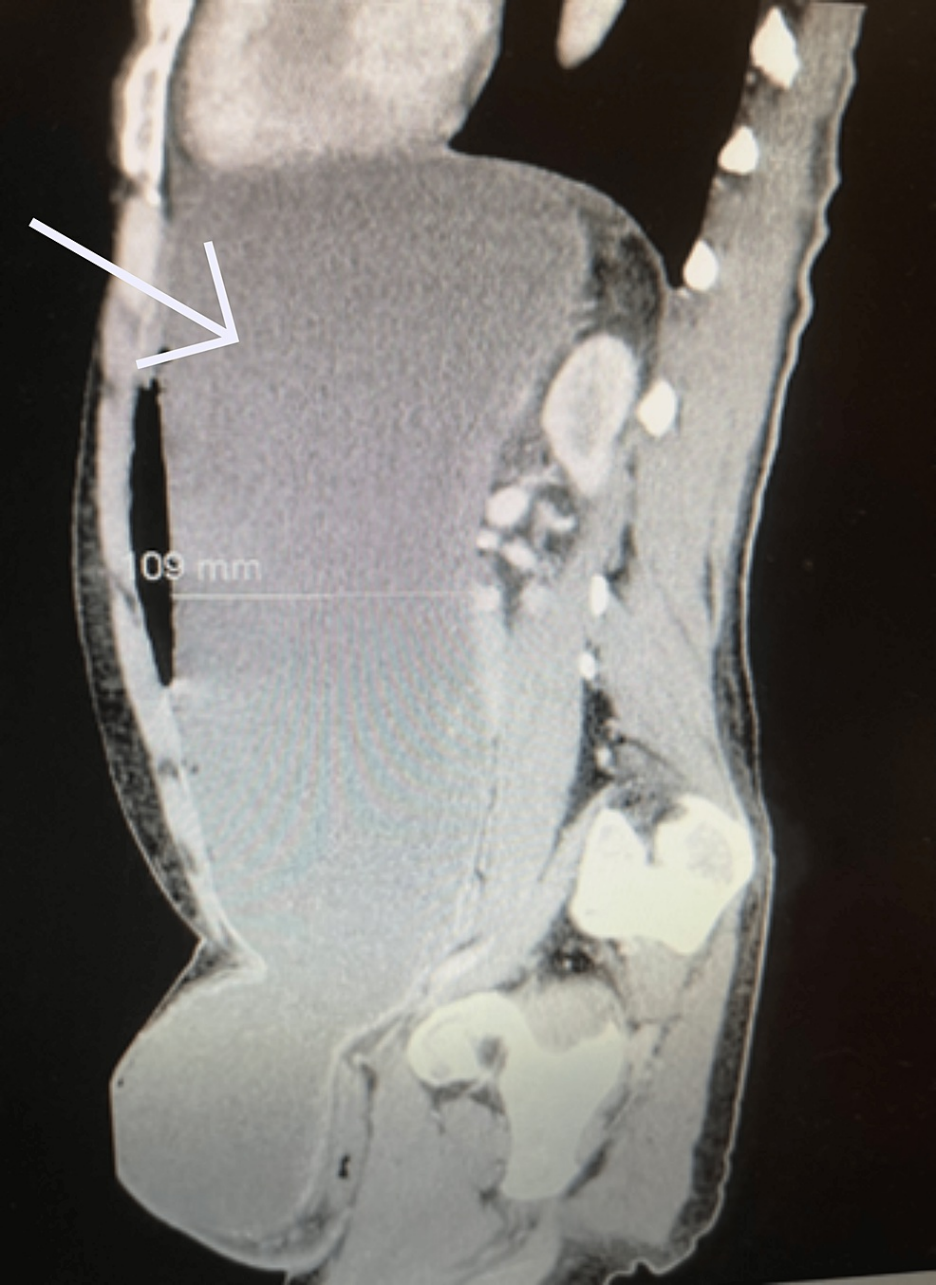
Computed tomography of abdomen and pelvis sagittal cross section showing dilated stomach extending into the left inguinal hernia. Arrow pointing toward dilated stomach measuring 109 mm in depth.
